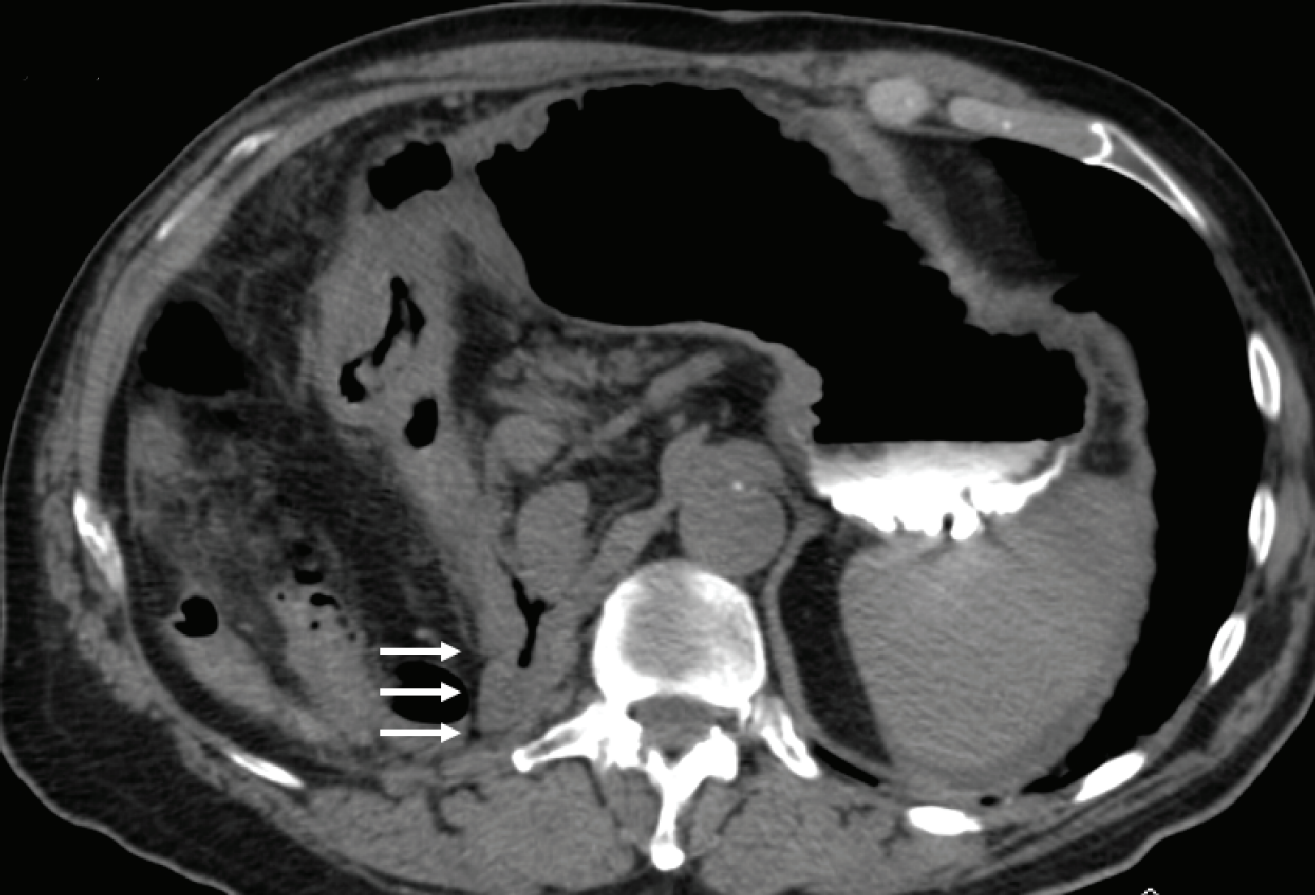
Multi-detector computed tomography (MDCT) multi-planar reconstruction shows herniation of the duodenum into the renal space (white arrows).
